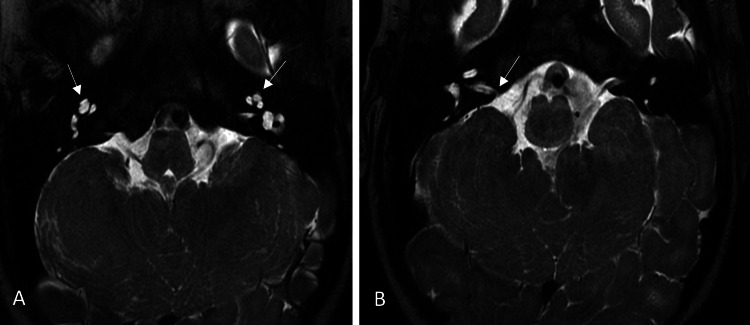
A: Isolated cochleae bilaterally with the absence of the cochlear canals (arrows). B: Narrowing of the right internal acoustic meatus with stenosis at the porus acusticus internus (arrow). A and B: Axial T2-weighted high-resolution images of the internal acoustic meati and inner ears.
